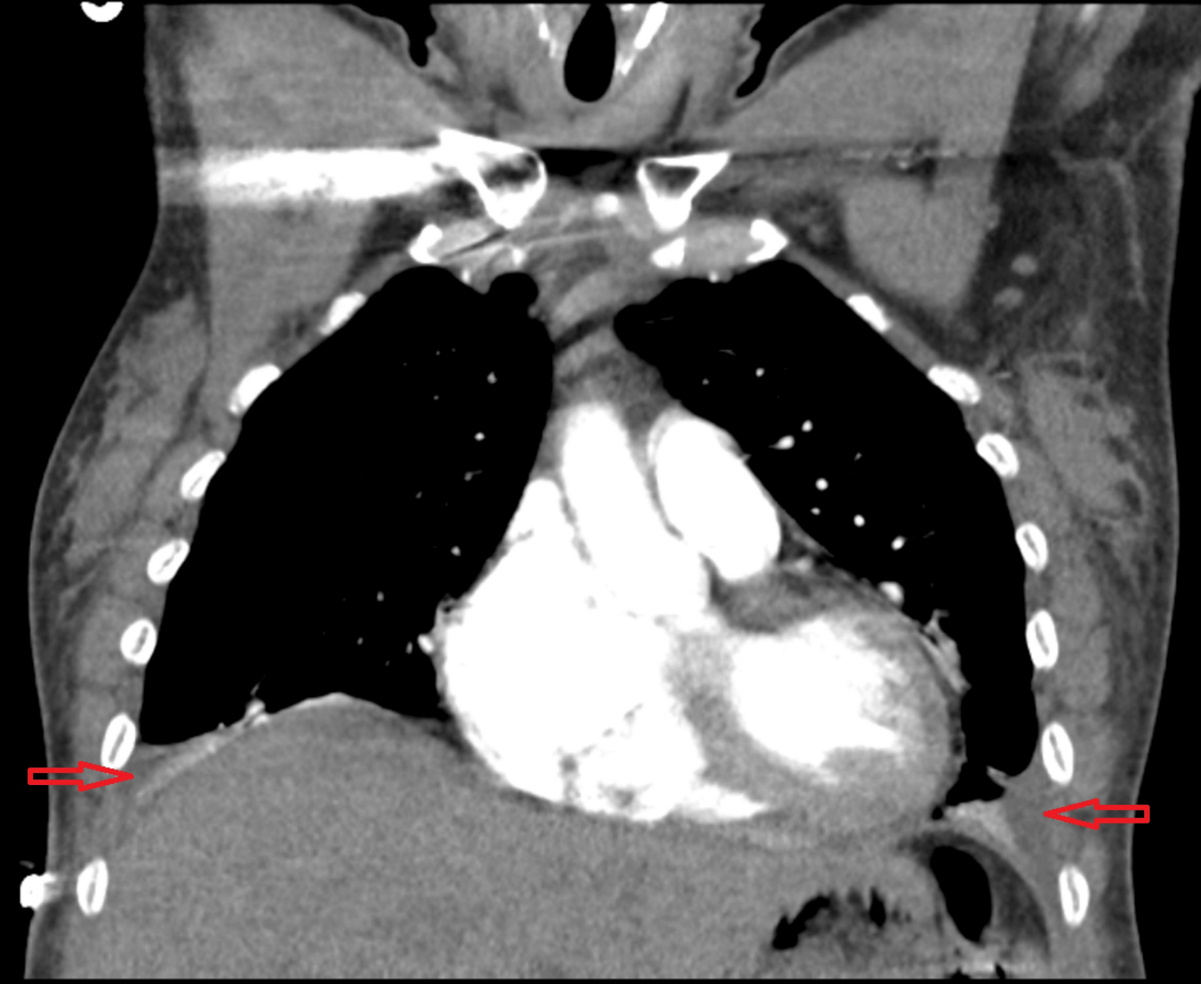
Coronal view of computed tomography (CT) angiogram of the chest showing moderate bilateral pleural effusions on the day of admission (shown by red arrows).
